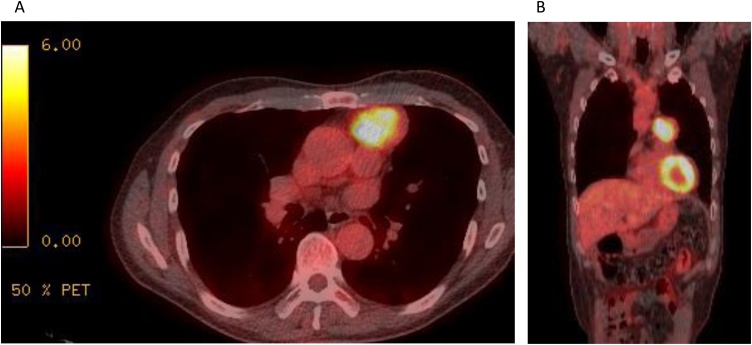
(A–C) Positron emission tomography/computed tomography. The thymic mass showing marked uptake of 18F-fluorodeoxyglucose; the early maximum standardised uptake value (SUVmax) of the mass was 9.8.
